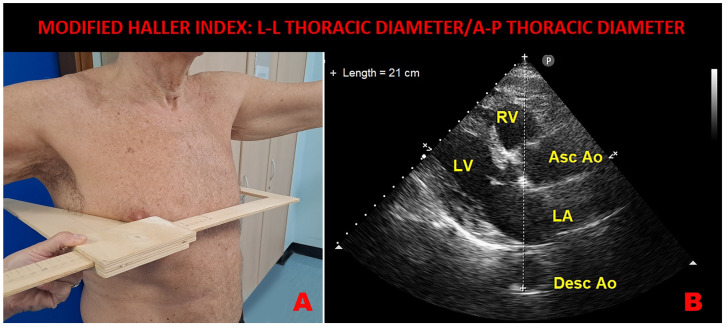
Example of modified Haller index assessment in an elderly male with circular transverse thoracic shape section, affected by asymptomatic persistent atrial fibrillation. (Panel (A)): L-L thoracic diameter, measured with the patient in the standing position and with open arms by using a rigid ruler in centimeters coupled to a level (the measuring device) placed at the distal third of the sternum. (Panel (B)): A-P thoracic diameter, obtained from the echocardiographic parasternal long-axis view, as the distance between the true apex of the sector and the posterior wall of the descending thoracic aorta, visualized behind the left atrium. Ao, aorta; A-P, antero-posterior; Asc, ascending; Desc, descending; LA; left atrium; L-L, latero-lateral; LV, left ventricle; RV, right ventricle.
